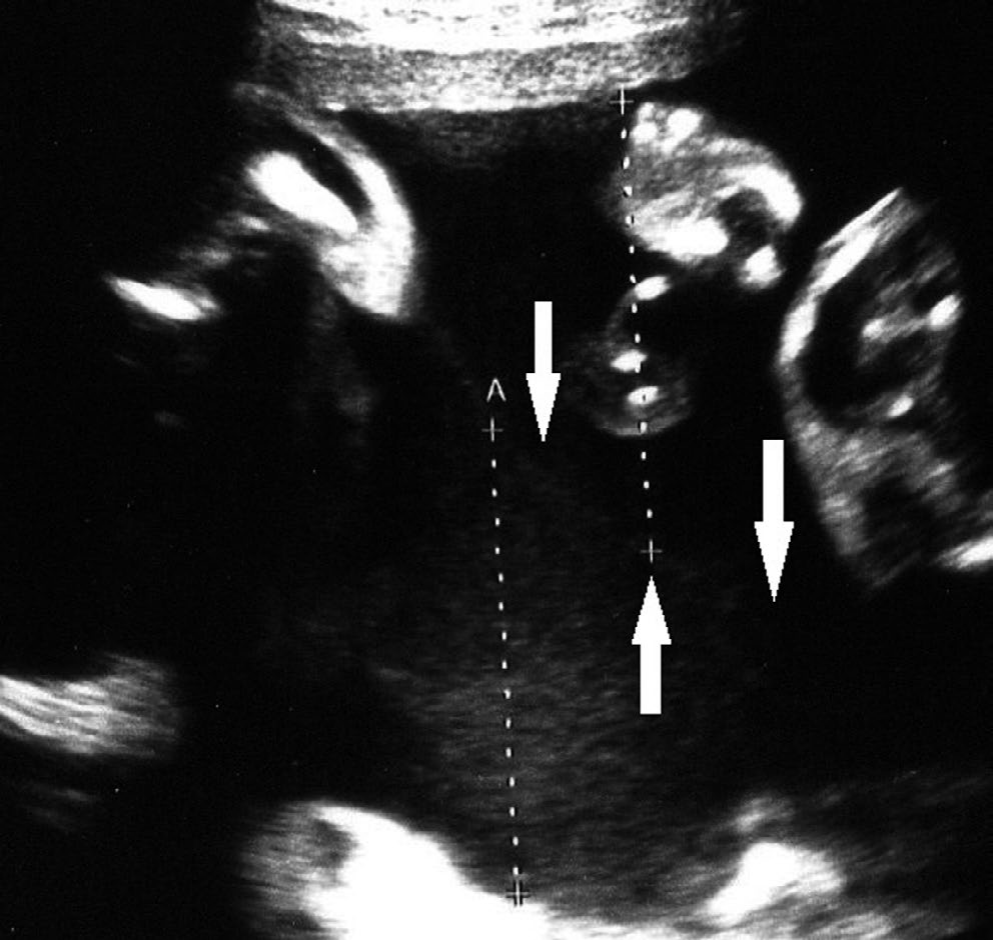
The presence of dividing membrane demonstrated between the twins by ultrasonography at 32 wkeeks of gestations (white arrows)
